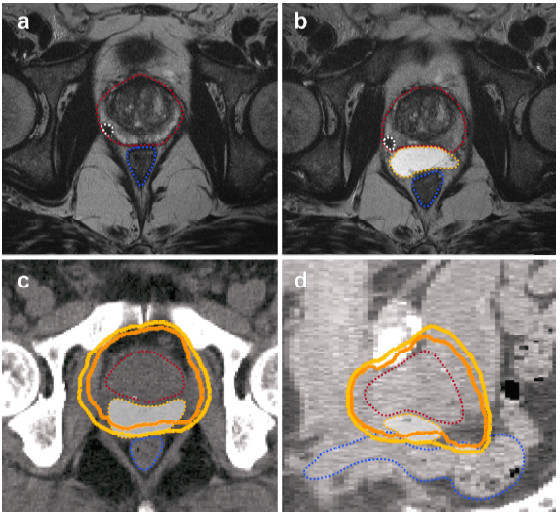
Example of MRIs (T2 TSE) performed before (a) and after (b) injection of a hydrogel spacer. The respective plan in axial (c) and sagittal view (d) with 70 Gy (light orange) and 74 Gy (orange) isodoses shows the rectal sparing with the use of the hydrogel spacer. Prostate (red), tumor (white), rectum (blue) and hydrogel spacer (yellow, white shading in CT scans) are indicated. *Abbreviation:* TSE=Turbo spin echo.
